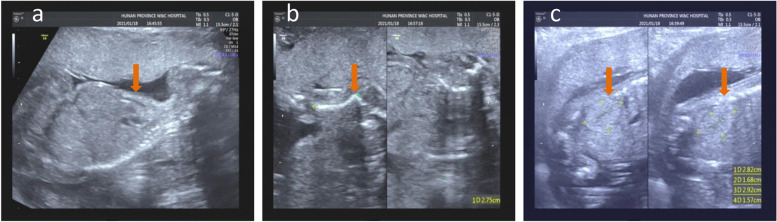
Ultrasound features of the fetus in patient 3. Narrow thorax (a), short bell-shaped ribs (b), and enlarged kidneys and enhanced renal parenchymal echo (c) are indicated with red arrows
